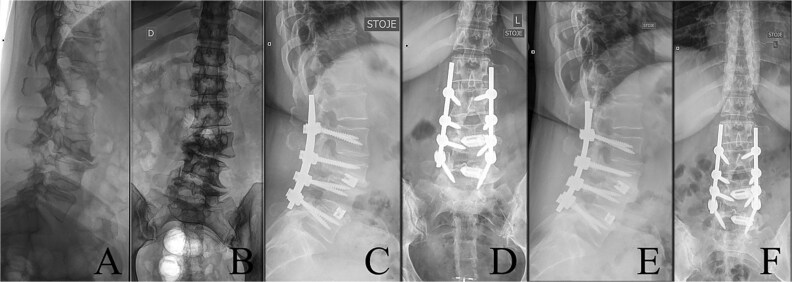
Last preoperative X-ray, lateral (A) and anteroposterior view (B). After 10 weeks of follow-up; lateral (C) and anteroposterior view (D). After 1 year of follow-up; lateral (E) and anteroposterior view (F).
